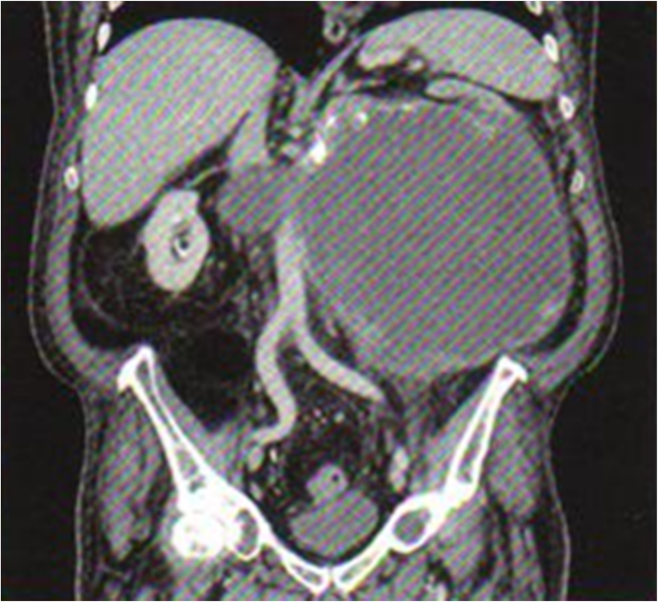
Abdominal CT sowing the huge renal tumor.
